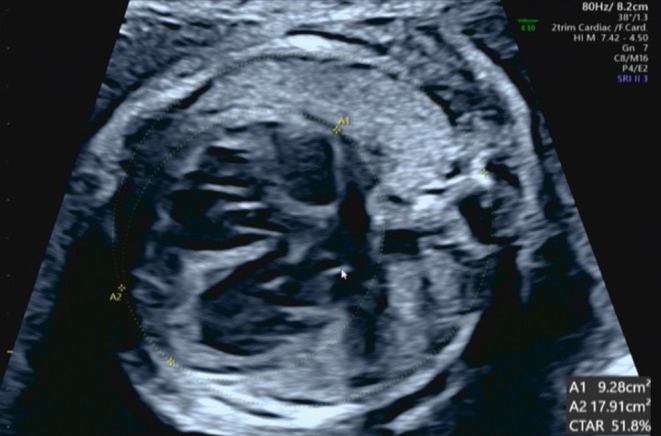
Fetal ultrasound at 29 weeks showing marked cardiomegaly and left ventricular noncompaction.
